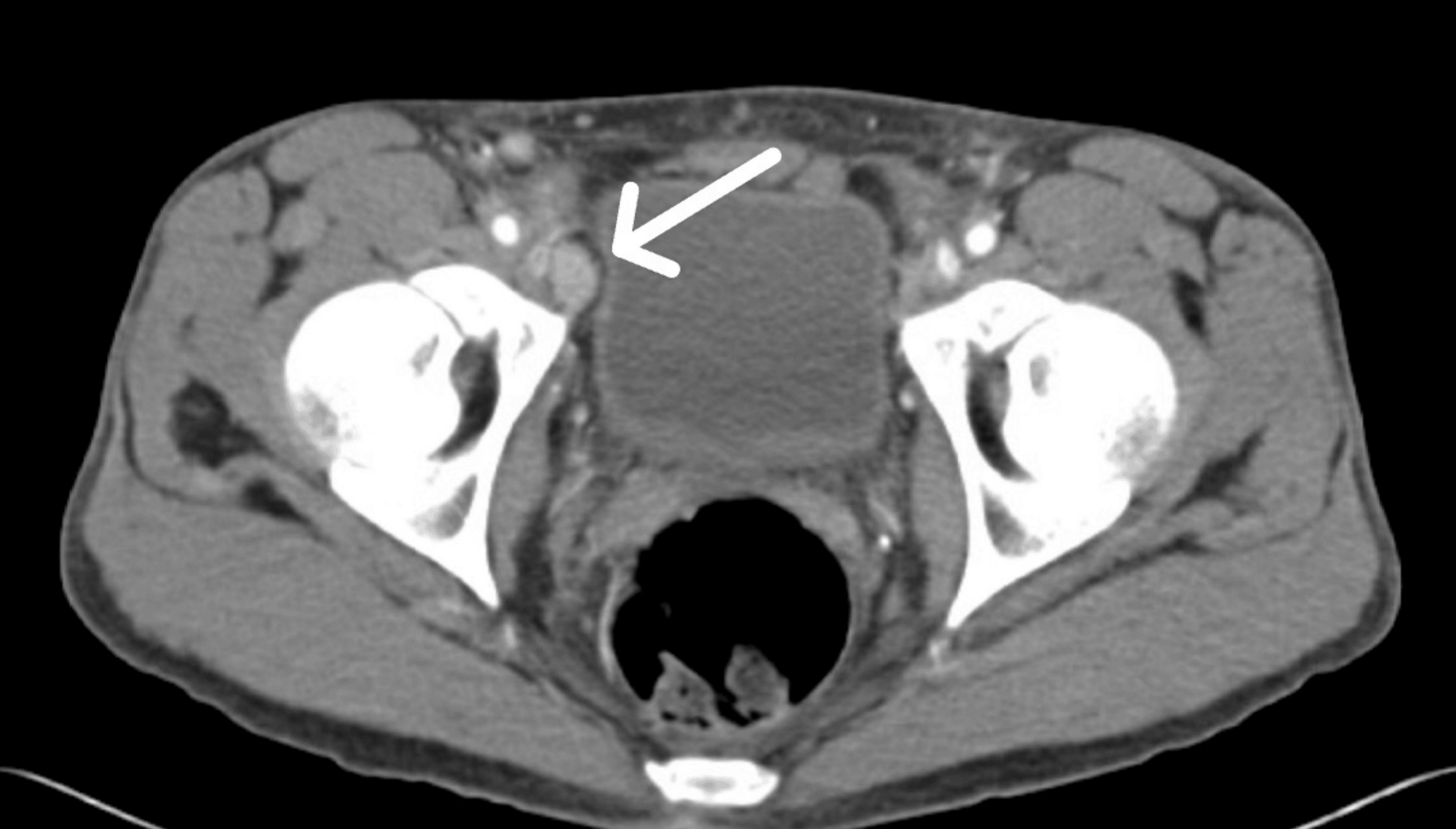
CT scan of the pelvis demonstrating right external iliac lymph node enlargement (arrow)
